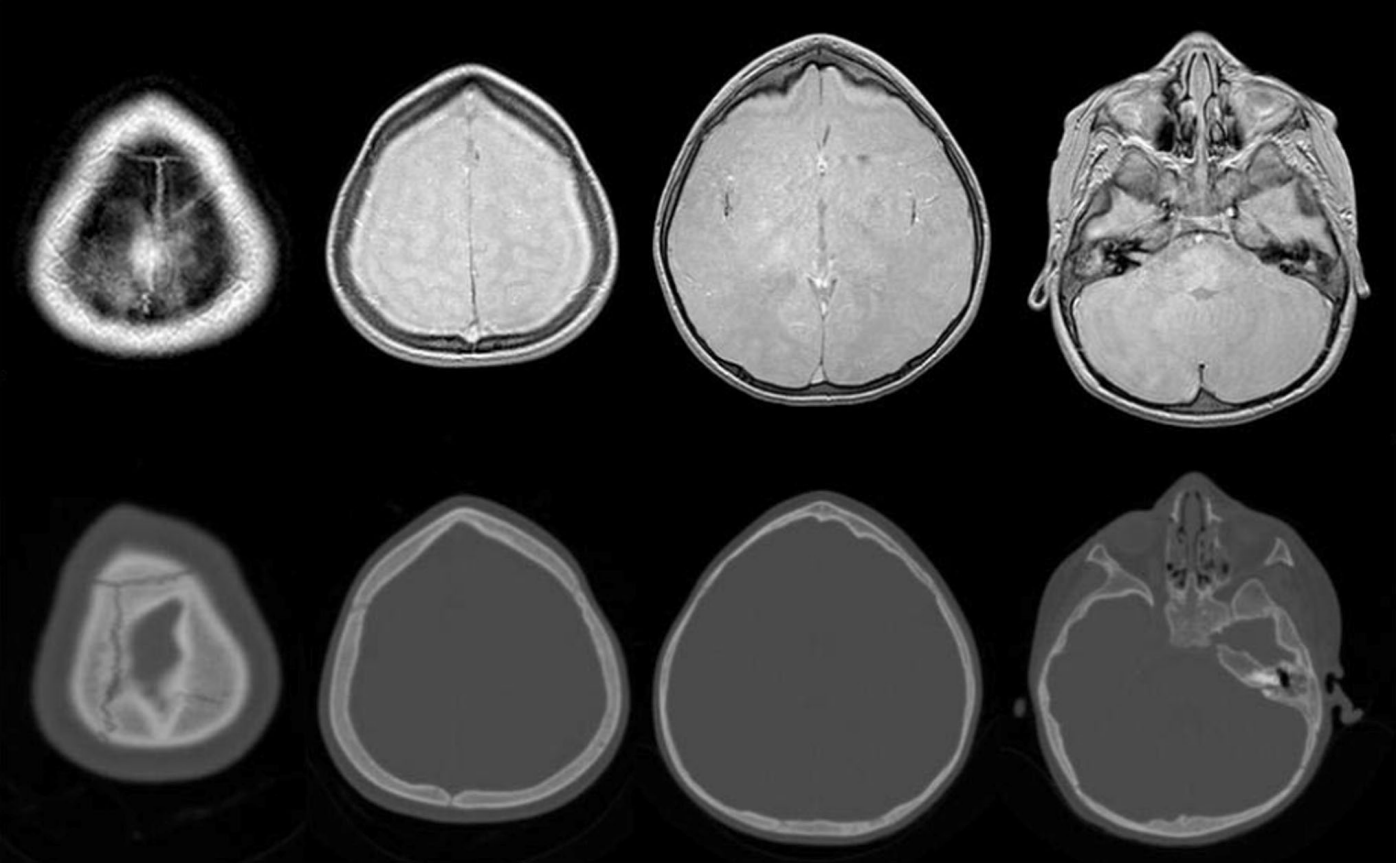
Axial GRE-BB (top row) and CT (bottom row) in a 17-month-old child with metopic synostosis. Note the trigonocephaly and absence of metopic suture, but the normal coronal sutures
